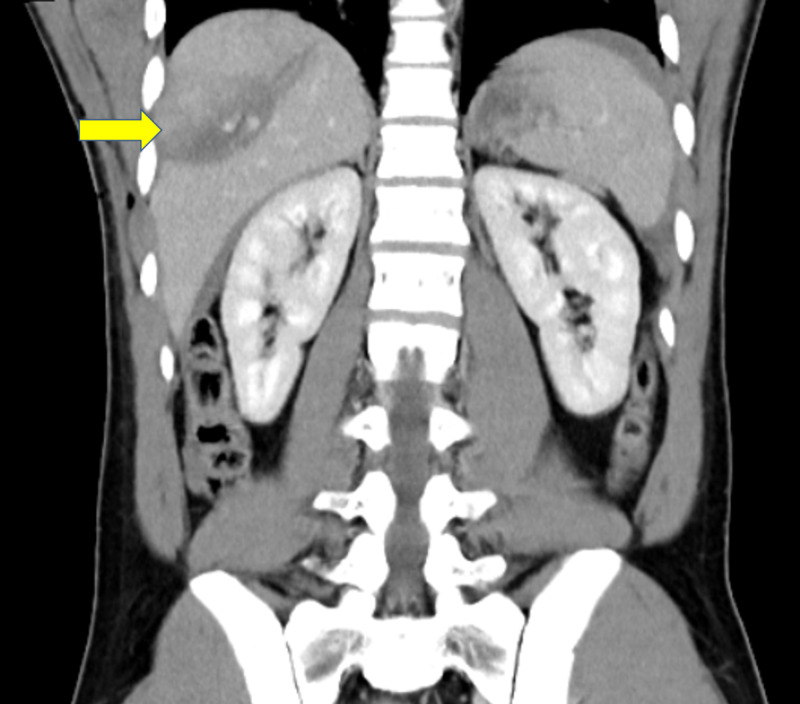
Abdomen CT, coronal view, showing grade 3 hepatic laceration after stab (yellow arrow)
